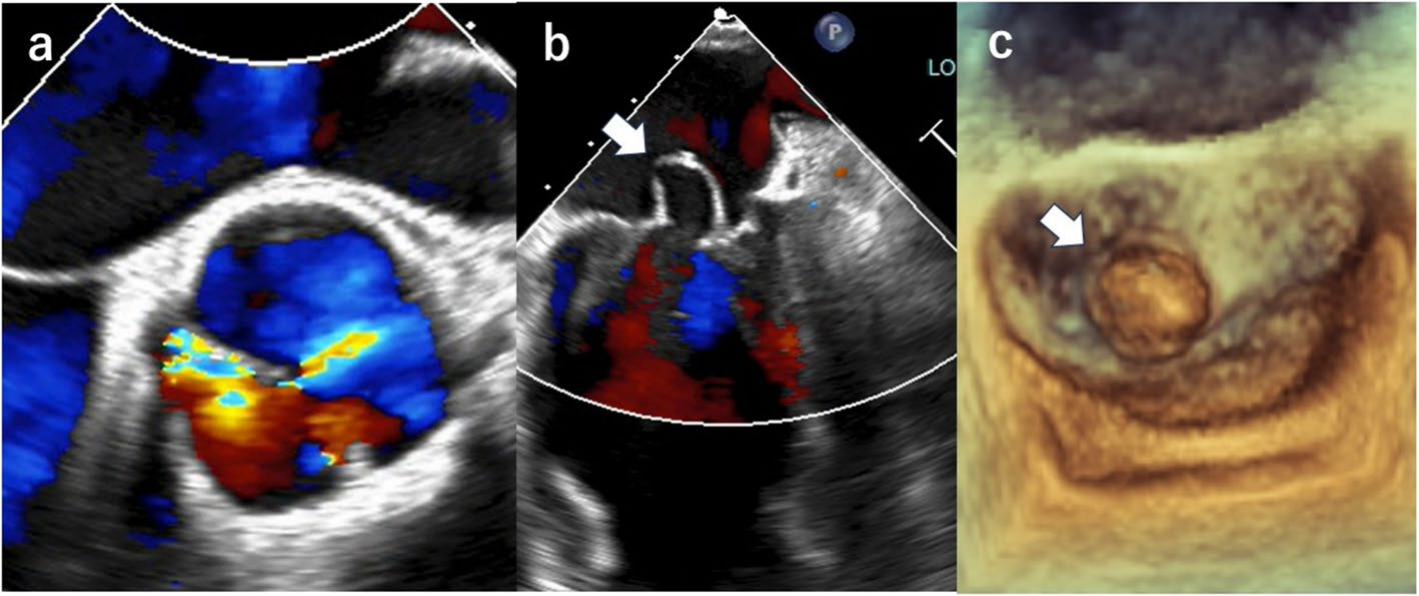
Transesophageal echocardiograms showing a Sievers Type I bicuspid aortic valve with fused left and right coronary cusps and aortic regurgitation (a) and an approximately 1.2-cm aneurysm of the anterior mitral valve leaflet (white arrow) (b and c)
